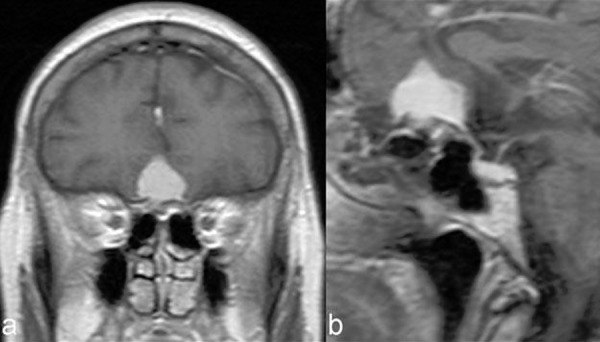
Picture showing a preoperative MRI scan showing the case of a planum sphenoidale meningioma.
